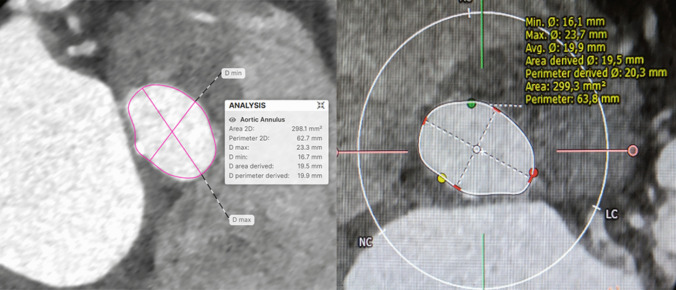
Measurement of the aortic annulus by the artificial intelligence-based platform (left picture), Measurement of the aortic annulus by conventional semi-automatic post-processing CT software (right picture)
